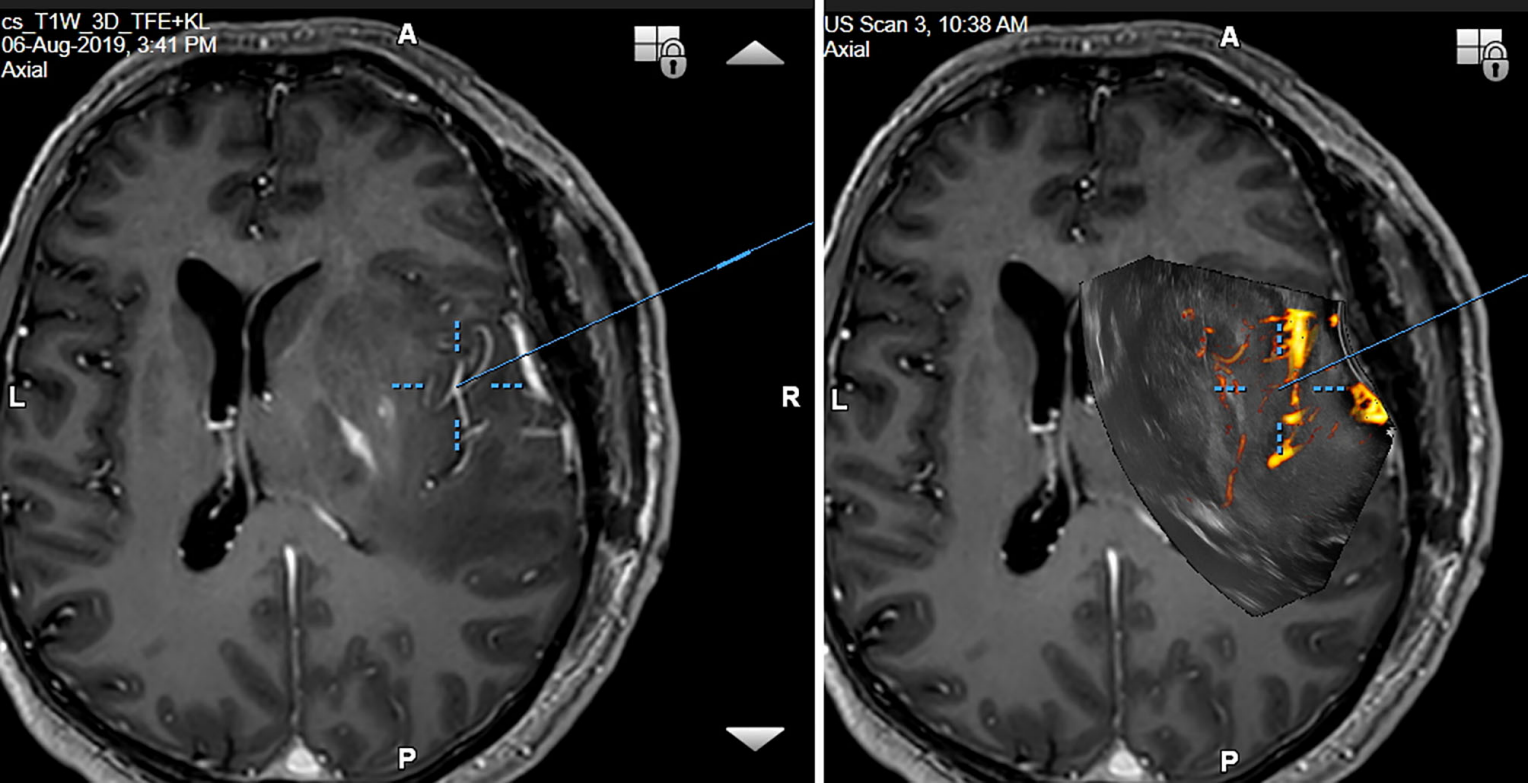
Right-sided temporo-insular glioma (reoperation after previous urgent brainstem decompression) (Left column) preoperative navigation 3D contrast-enhanced T1-weighted MRI sequence (3-Tesla MRI scanner) (Right column) pre-resectional 3D IOUS image fused with navigation MRI using a high-end navigated 3D IOUS system. Note the excellent visualization of perforating lenticulostriate arteries under the infiltrated right insula. 3D, three-dimensional; MRI, magnetic resonance imaging; IOUS, intraoperative ultrasound.
